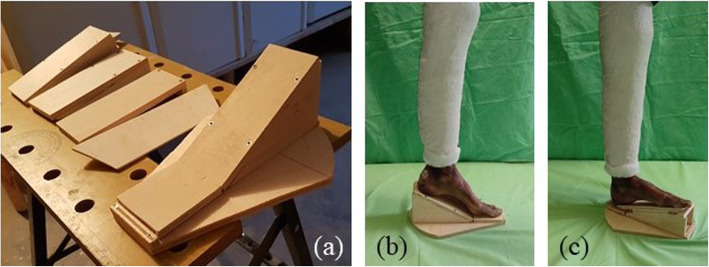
The wedges employed to impose foot posture during CT scanning (a) and their application in two cases: 30° plantar flexion (b) and a combination of 20° dorsi flexion and 10° supination (c)
